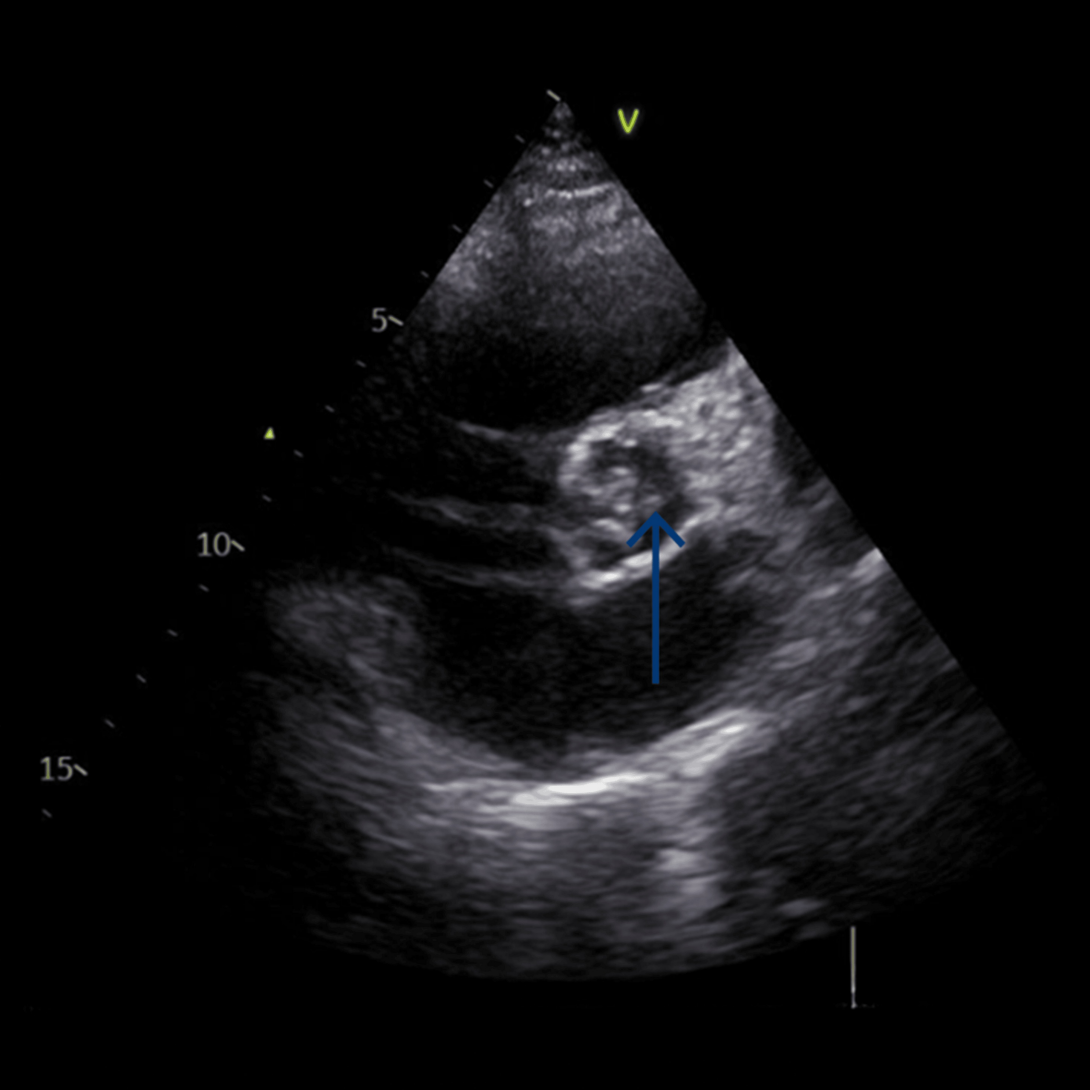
Transoesophageal echocardiography showing aortic valve vegetation.
